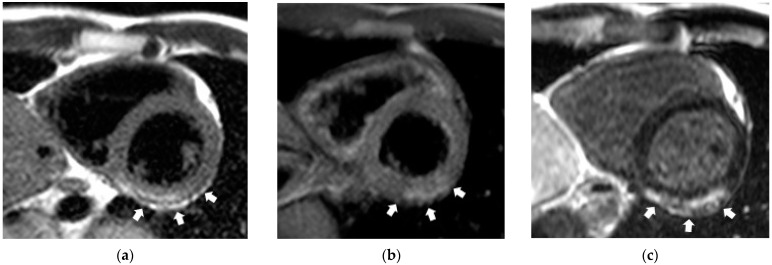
Contrast-enhanced cardiac magnetic resonance imaging in a patient with history of COVID-19 and three Lake Louise criteria of myocardial inflammation: edema, hyperemia, and fibrosis. White arrows indicate (a) edema (T2-weighted image), (b) hyperemia of the left ventricular inferior wall (early post-contrast enhancement on T1-weighted image), and (c) fibrosis (late contrast enhancement in inversion recovery mode).
